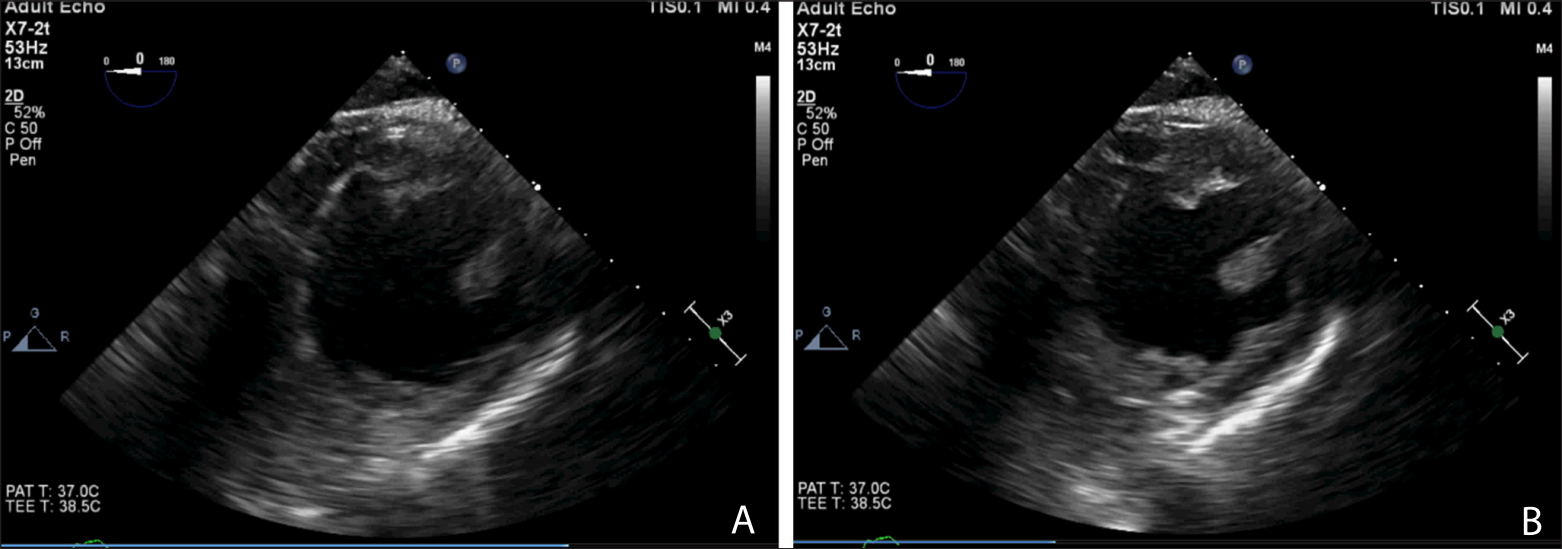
(A) Transgastric transesophageal echocardiography (TEE) short axis view of a failing left ventricle at end systole. (B) TEE short axis view of a failing left ventricle at end diastole. Photo credit Chandrika Garner, MD, Atrium Health Wake Forest University Medical Center
